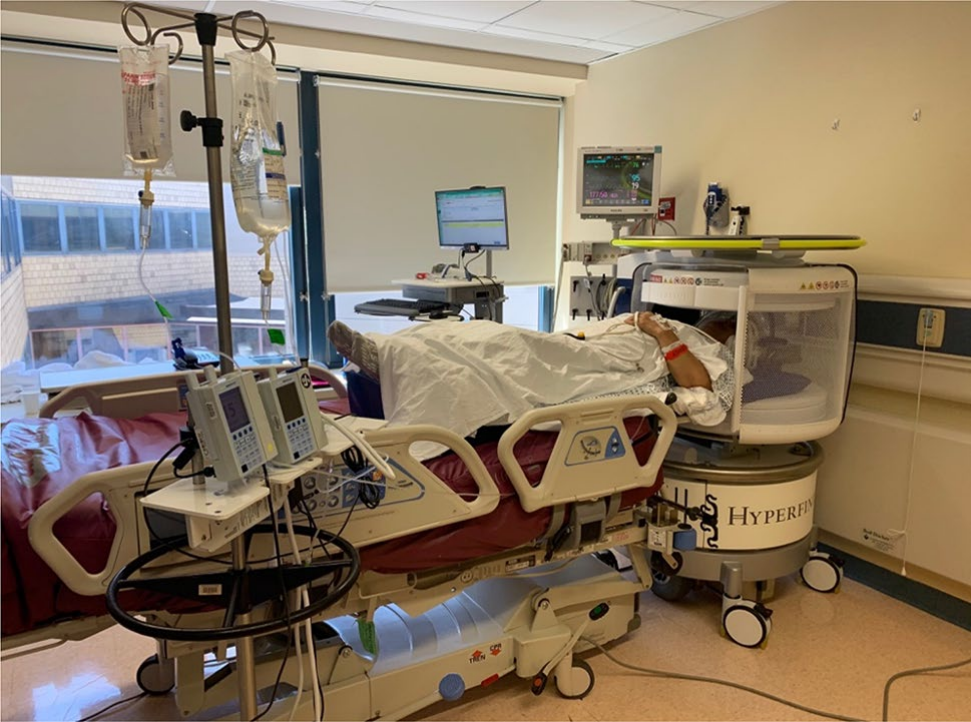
0.064 T Portable MRI scanner in an intensive care unit room. Low-field portable MRI exams were performed in the presence of operational intensive care equipment. The portable MRI operator and bedside nurse were able to remain in the room during scanning. All portable MRI images were available for real-time viewing on an iPad as each sequence was acquired and processed.
